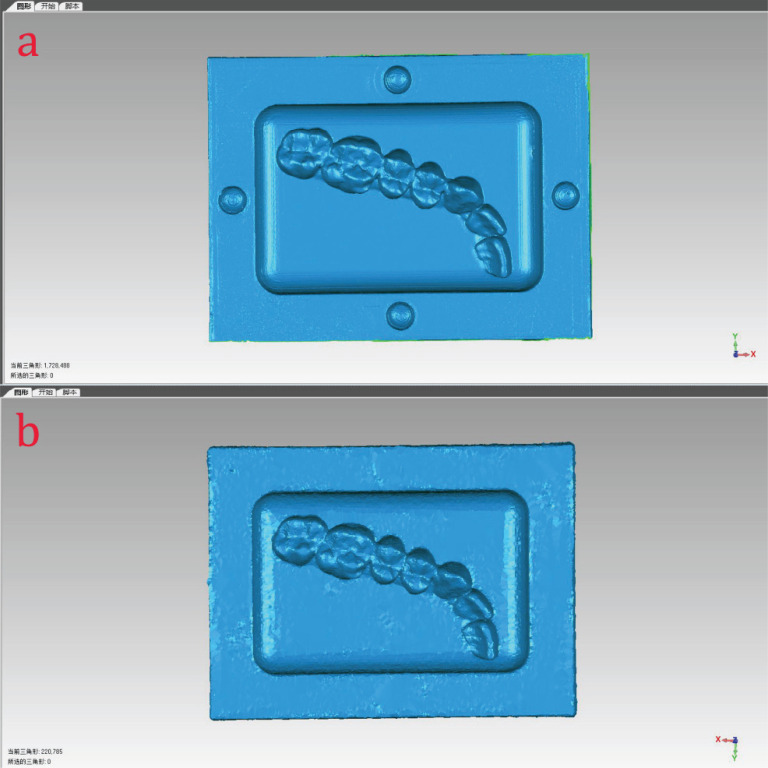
Structured light scanned model (a) and reconstructed CBCT model (b).
